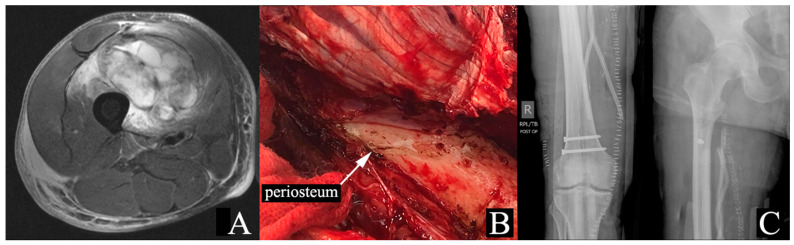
Seventy-one-year-old male with right thigh pleomorphic rhabdomyosarcoma. The patient was treated with 50 Gy of neoadjuvant RT. (A) MRI STIR sequence demonstrating proximity of the tumor to the femur, without invasion. (B) Intraoperative image showing periosteal stripping of the femur. (C) Plain radiographs displaying after periosteal stripping and RT. MRI: magnetic resonance imaging; STIR: Short Tau Inversion Recovery; RT: radiation therapy.
